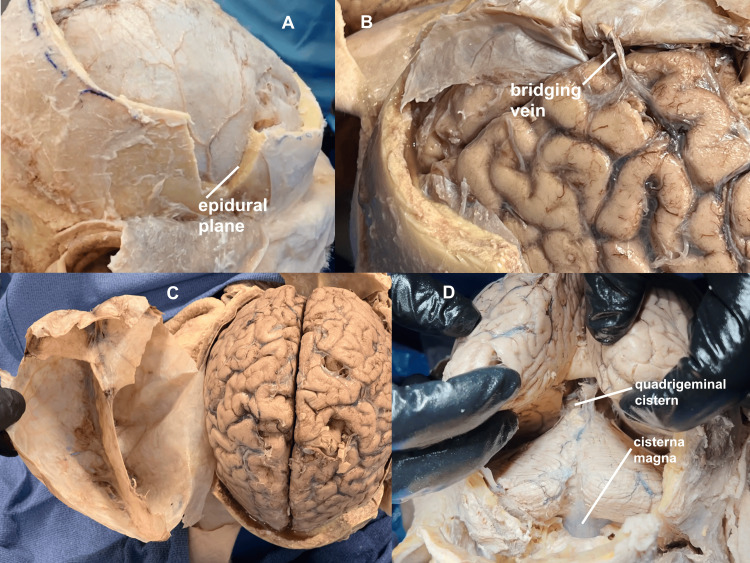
Optimized learning opportunities The technique allows for a number of learning opportunities during the craniotomies. (A) The pterion can be removed individually or with a wide strip of the additional calvarium, demonstrating the middle meningeal artery and the tight epidural plane where the bleeding would accumulate, explaining the time such periosteal elevation would take, hence the lucid interval, as well as the lens or biconvex shape of the epidural haemorrhage on a CT scan. (B) The bridging veins can be appreciated as the source of a subdural bleed, with the broad subdural space explaining the crescent nature of a subdural haematoma on a CT scan. (C) With the dura's integrity maintained, the various dural sinuses can be clearly visualized in three dimensions, associating them with the brain in situ. (D) Prior to breaking through the arachnoid layer, two major CSF cisterns can be appreciated: the Quadrigeminal cistern and the Cisterna magna.
